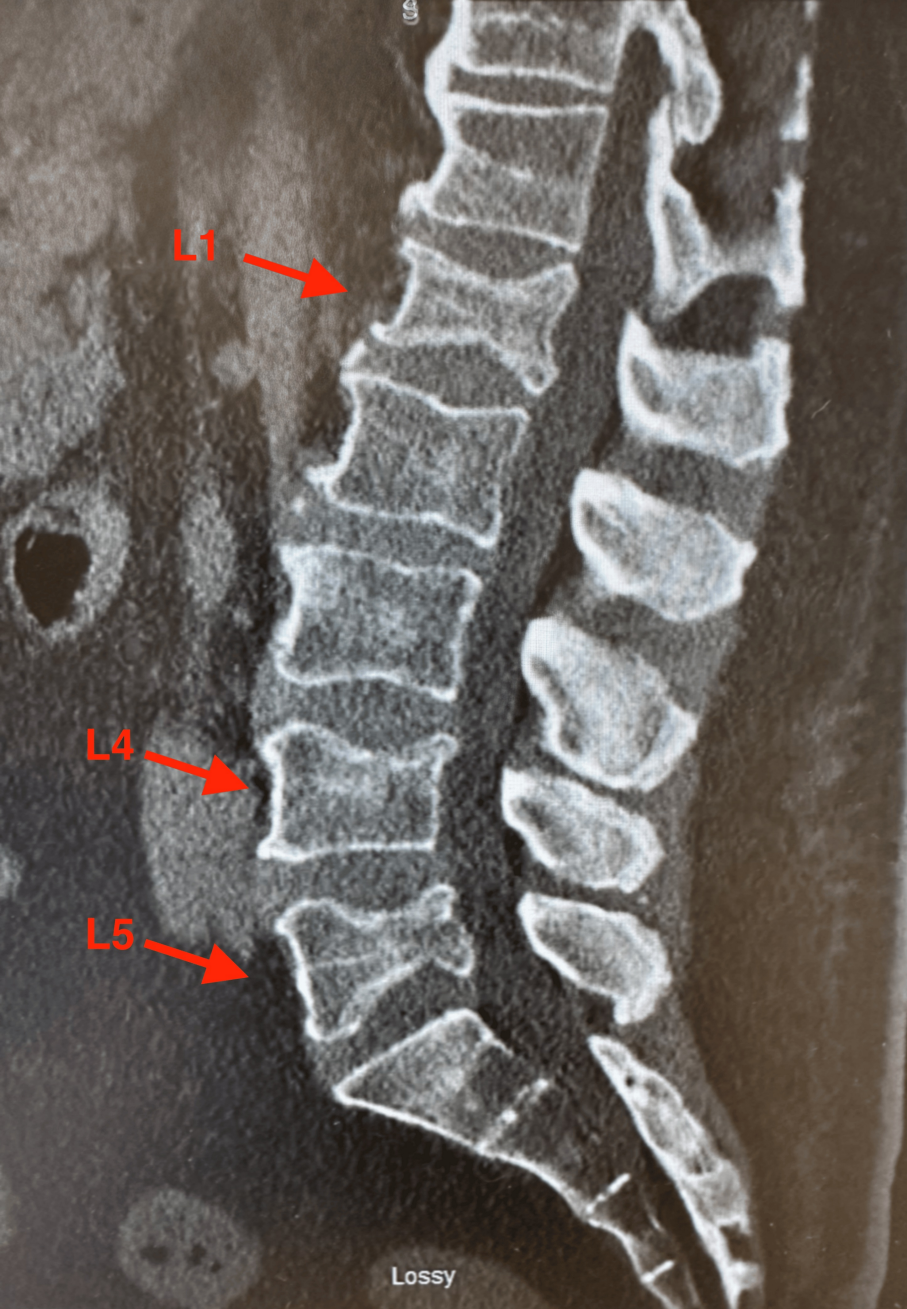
Sagittal CT scan of the lumbar spine demonstrating compression deformities involving L1, L4, and L5 (red arrows). The vertebral bodies show anterior wedging, decreased height, and cortical irregularity consistent with acute to subacute compression fractures. Image captured using a smartphone camera due to limitations in accessing downloadable files. All identifiable information was removed.
